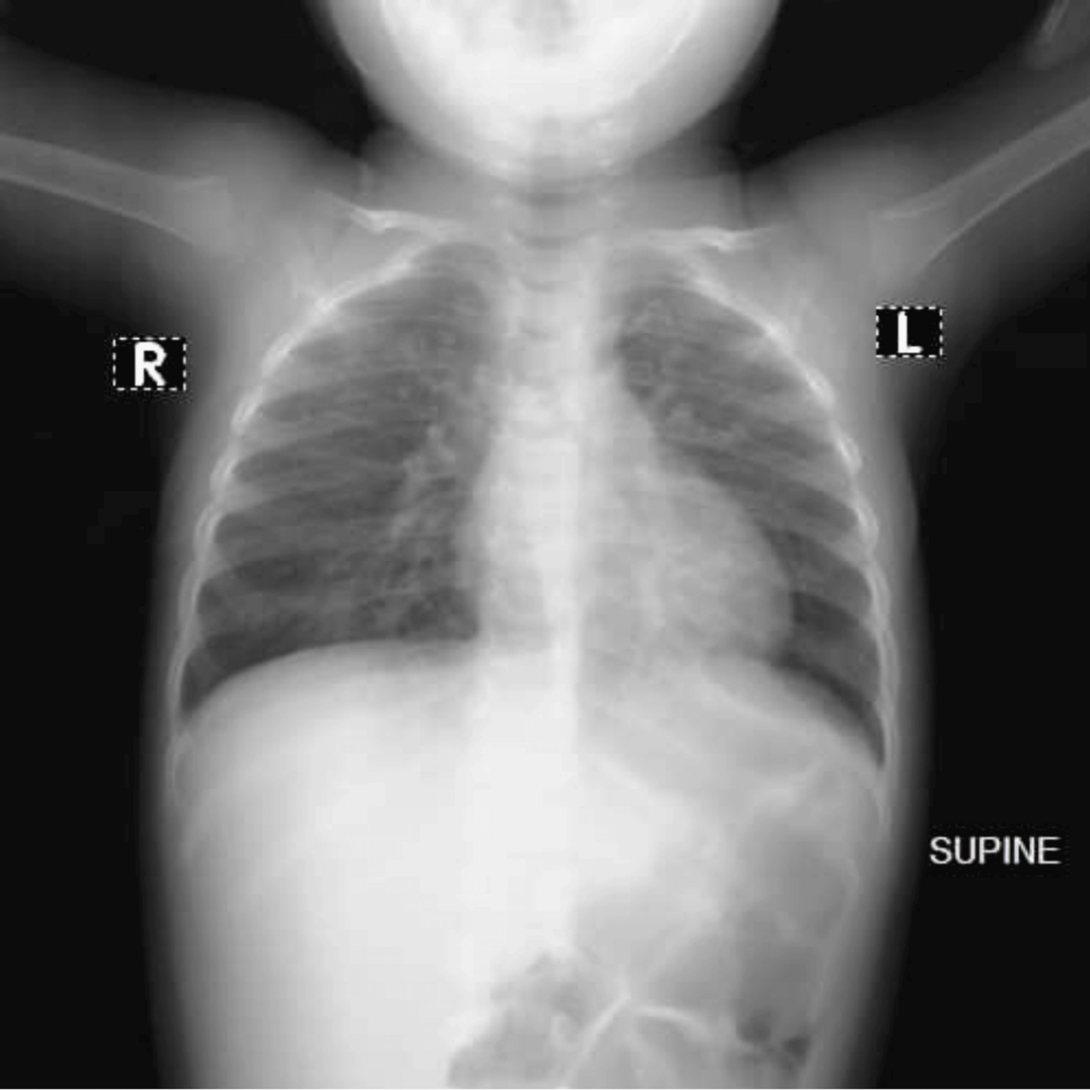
Normal AP supine chest X-ray AP: anteroposterior
